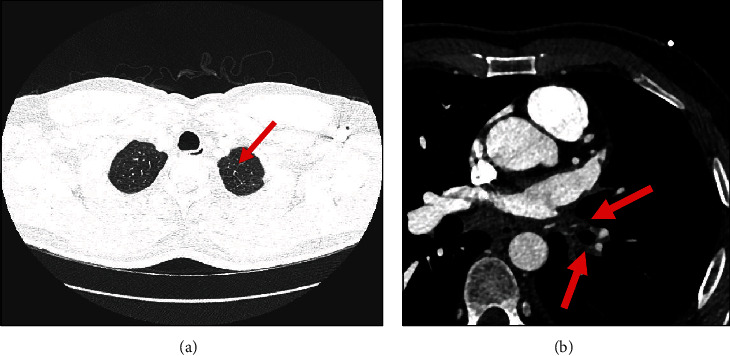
CTPA: (a) unilateral pulmonary edema with interlobular septal thickening (arrow); (b) Lt.PVsO (arrow). CTPA: computed tomography pulmonary angiogram; Lt.PVsO: left pulmonary vein occlusion.
